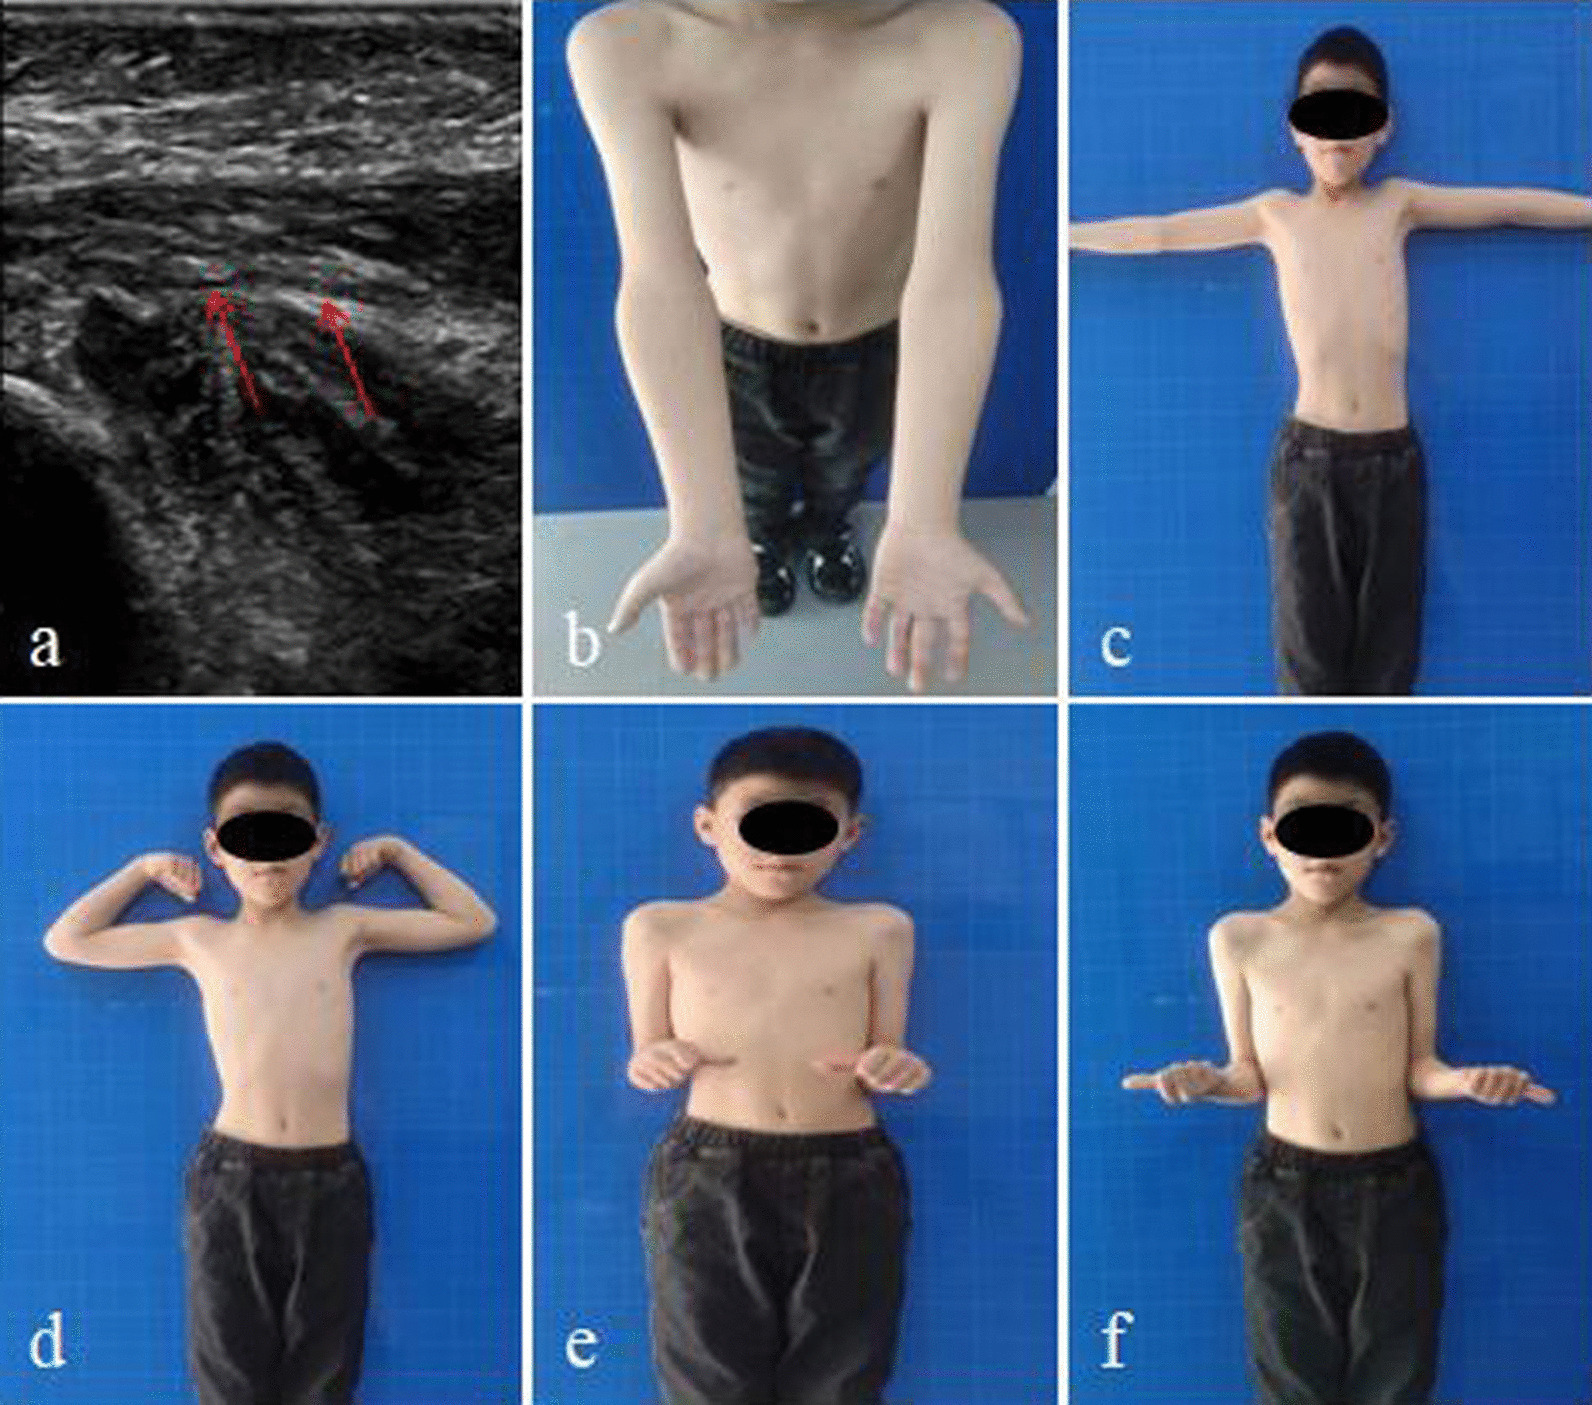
A 7-year-old child with radial nerve injury (red arrow): a uneven degeneration of the radial nerve was observed under ultrasonographic guidance; b–f the flexion, extension, pronation, and supination functions of the affected elbow joint at the last follow-up after recovery from acute Monteggia fracture in the child
